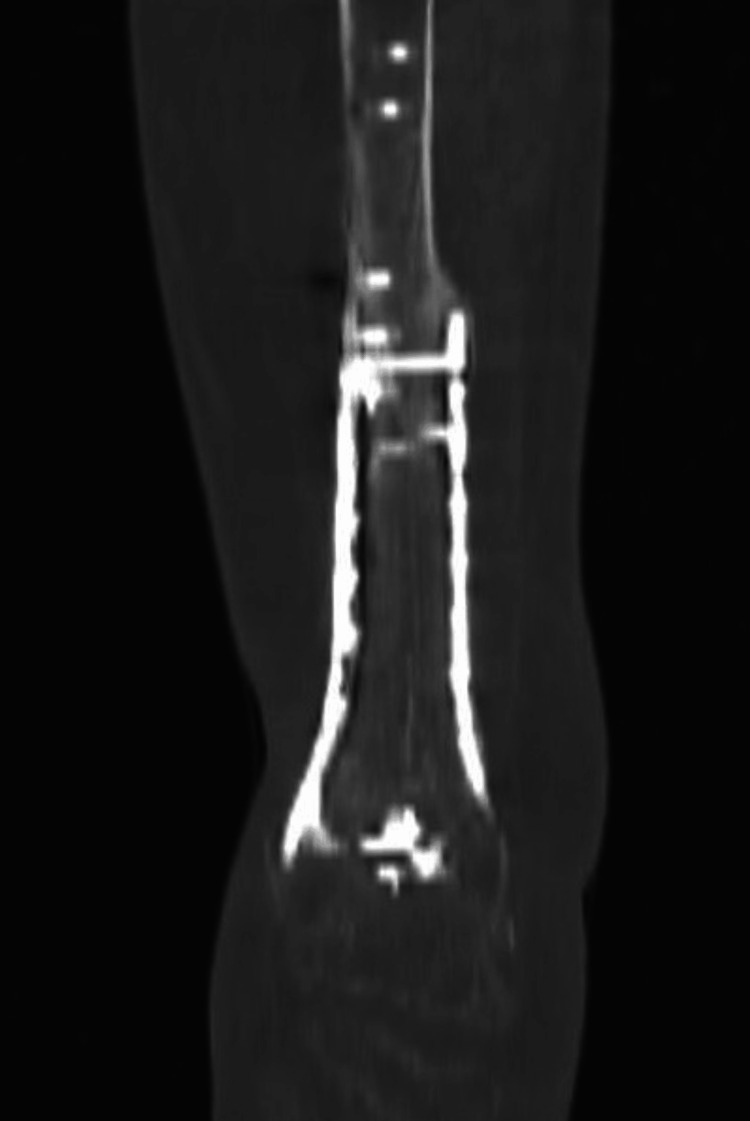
Case 2: Low-dose CT one year after the second-stage IMT IMT: Induced membrane procedure
